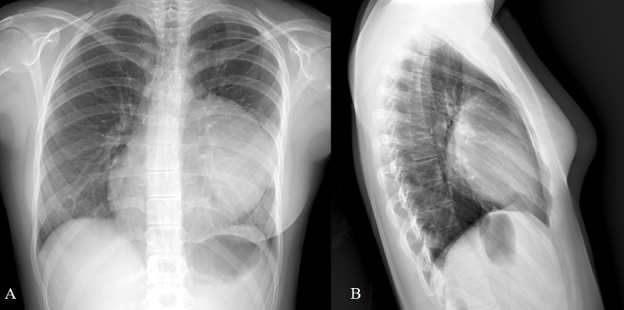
Figure 1: PA (A) and Lateral (B) chest X-rays showing a large homogeneous soft tissue mass.
